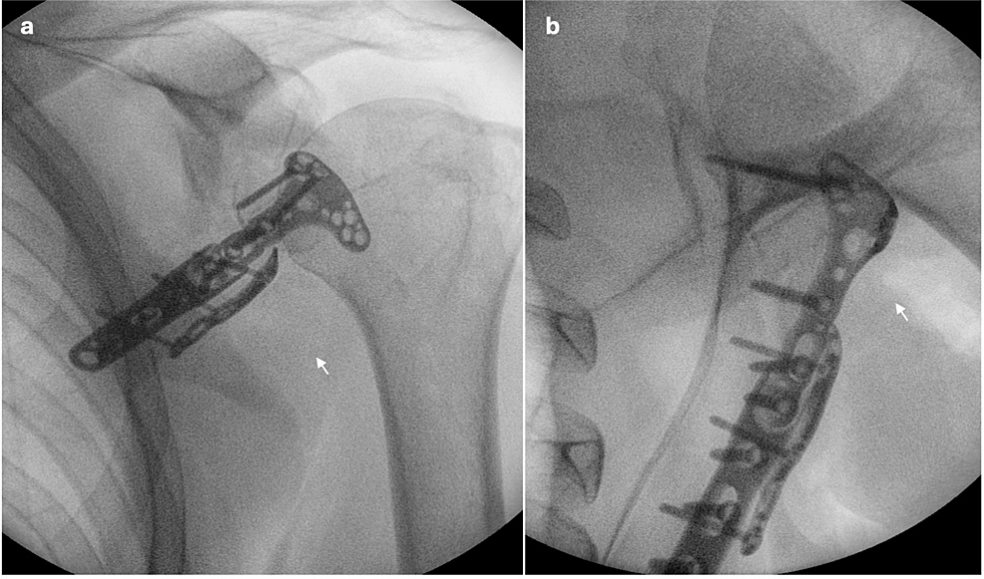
Intra-operative X-rays taken with an image intensifier machine. a. Posterior radiograph. b. Axial radiograph. Figures 8a-8b show fixation with a distal radius plate and screws provided good reduction of the fracture (white arrows).
